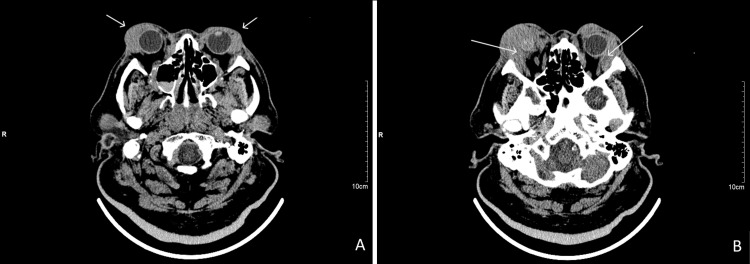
Non-contrasted axial-cut CT brain and orbit revealed bilateral extra-conal (A) and intra-conal (B) masses arising from the lacrimal gland with involvement of the extraocular muscles (A and B)
